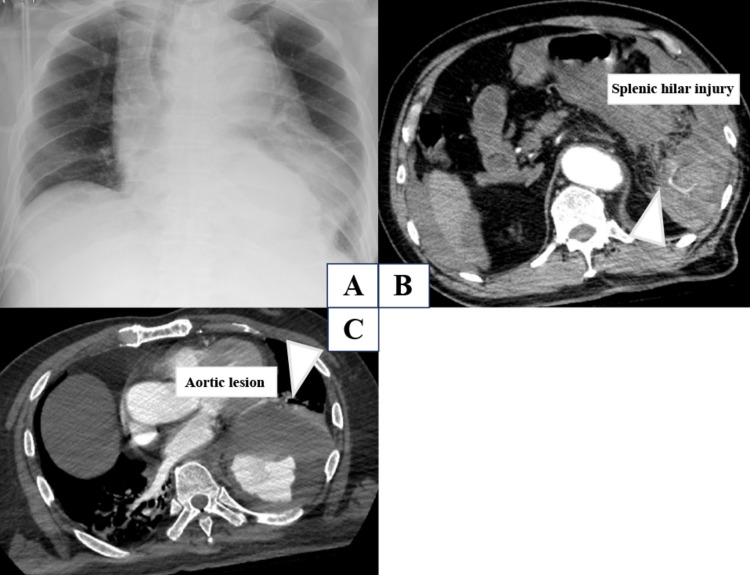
Imaging findings on admission. (A) Chest radiograph showing marked mediastinal widening. (B) Contrast-enhanced computed tomography showing splenic hilar injury with contrast extravasation (arrow) and hemoperitoneum. (C) Contrast-enhanced computed tomography demonstrating a traumatic subadventitial hematoma (arrow) in the descending thoracic aorta with aneurysmal morphology, consistent with grade II blunt thoracic aortic injury.
